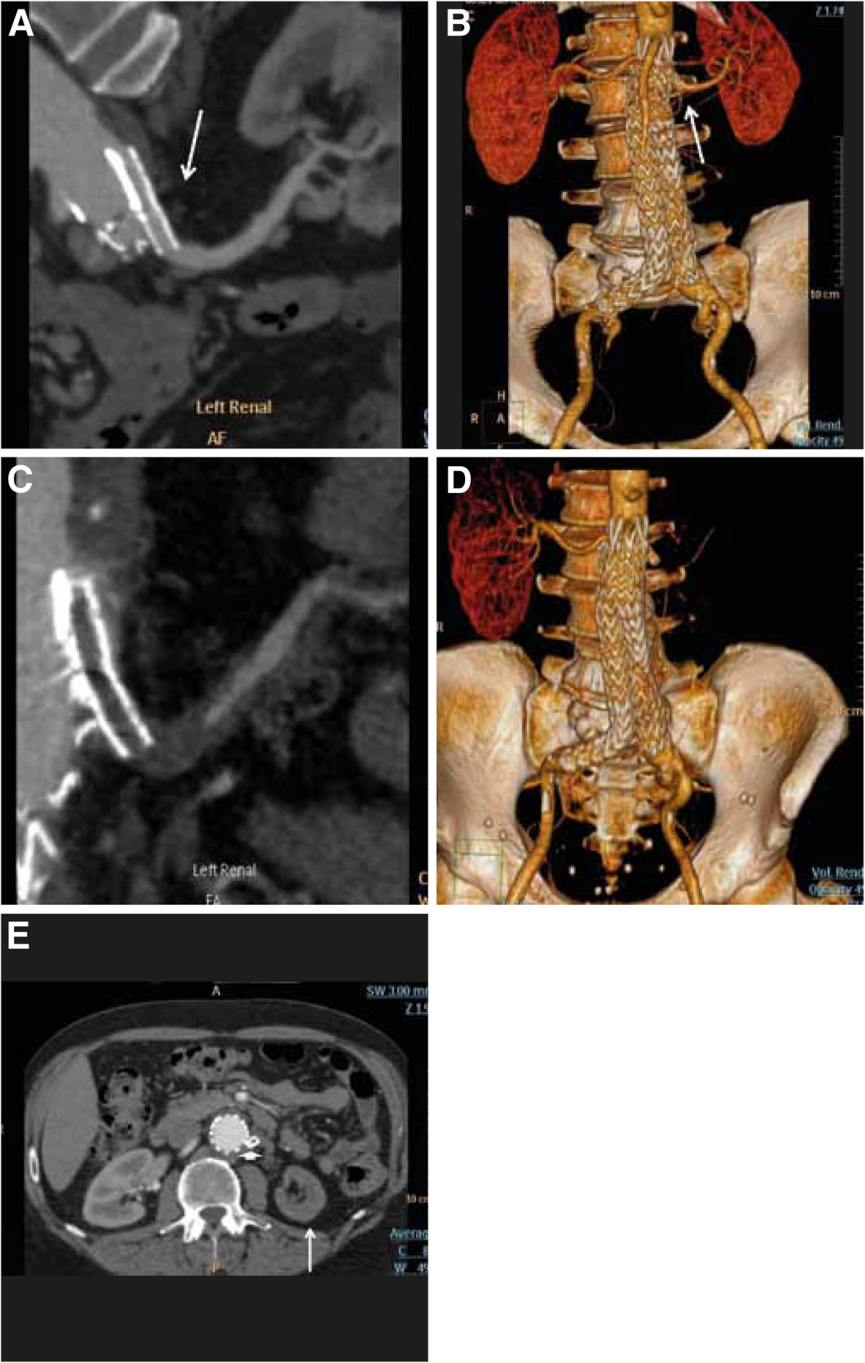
Abdominal CT-angiography (CTA) of a 64-year old patient after EVAR with placement of GC into the left renal artery. A-B. Five months before presentation, during routine post-operative follow up and C-E. At presentation with malignant renovascular hypertension; A. Curved Multi Planar Reconstruction (cMPR) image showing a patent CG (arrow). B. A volume rendering image showing patent aortic stent-graft and patent CG (arrow) with symmetric enhancement of the two kidneys. C. cMPR image showing occluded CG with near complete occlusion of the left renal artery. A trickle of contrast is demonstrated in a distal segment of the artery. D. A volume rendering image showing the occluded CG with lack of enhancement of the left kidney. E. Axial image showing the occluded CG (short arrow) and small, shrunken non enhancing left kidney (long arrow).
